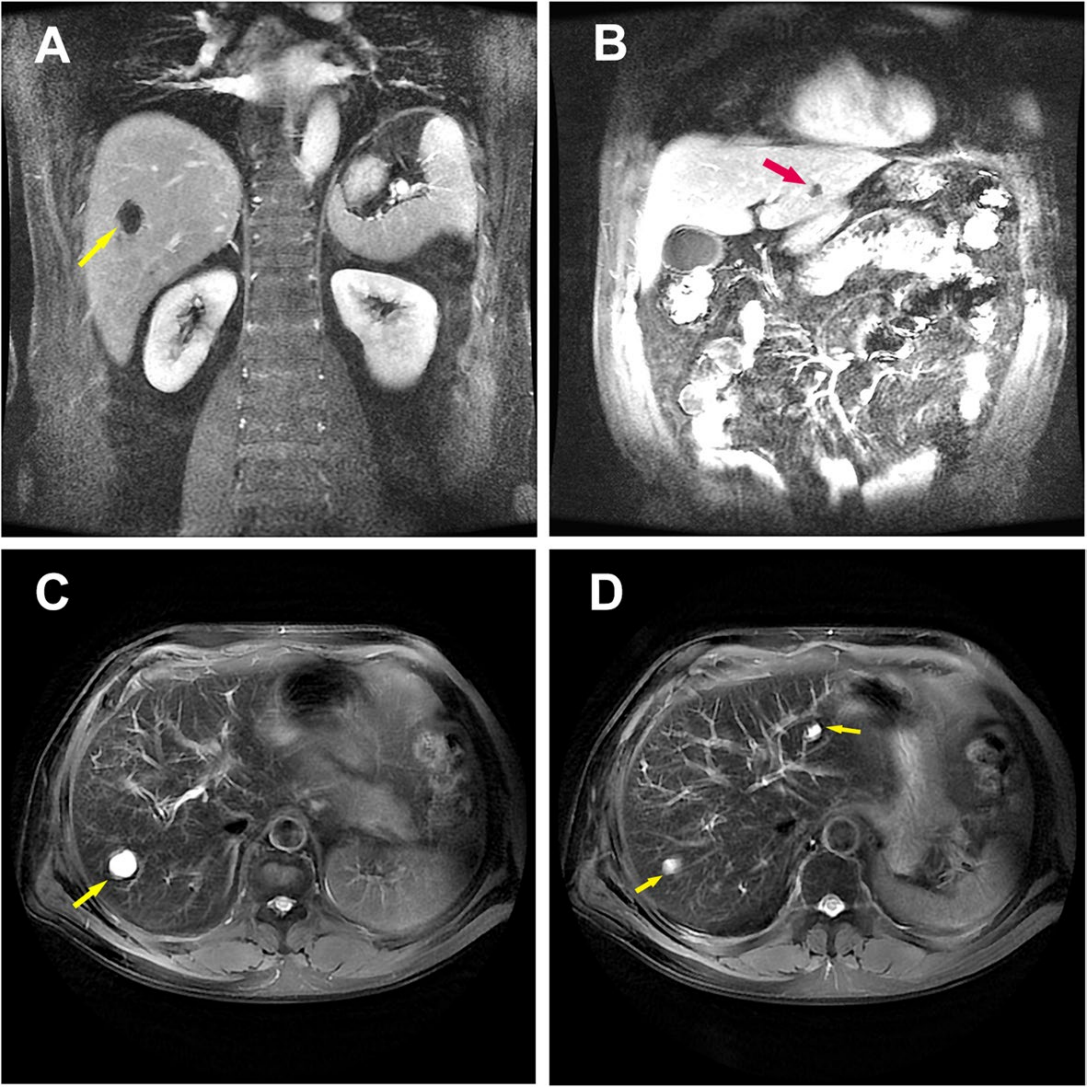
A-D Enhanced magnetic resonance imaging of the liver obtained at 1-month follow-up showed that lumpy abnormal signal (*arrow*) was observed in the right posterior lobe of the liver, about 28 × 31 mm in size, which was reduced compared with that of the previous one
